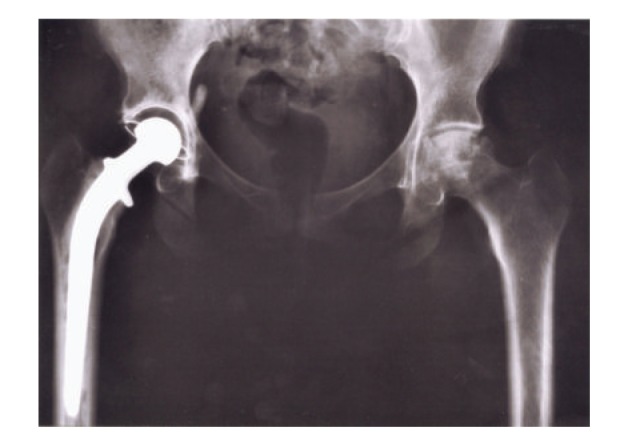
Hip X-ray image showing total replacement at left (right side) (from Wikipedia).
